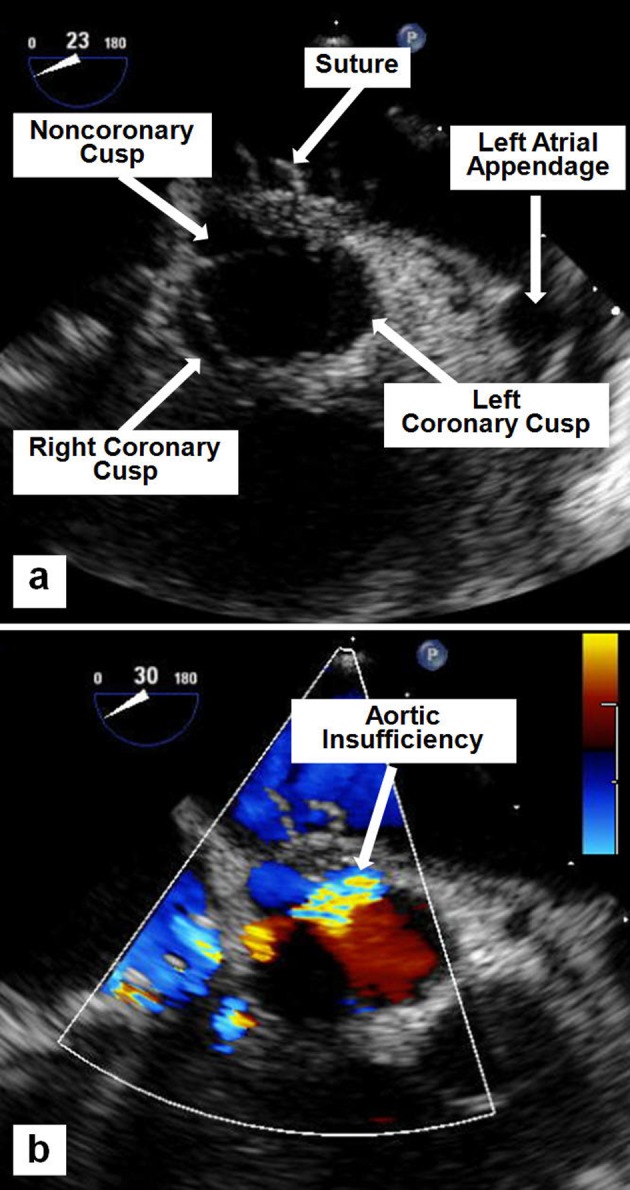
Mid-esophageal TEE images showing a suture tethering the non-coronary cusp (a), and the resulting moderate-to-severe AI by color flow Doppler (b).
